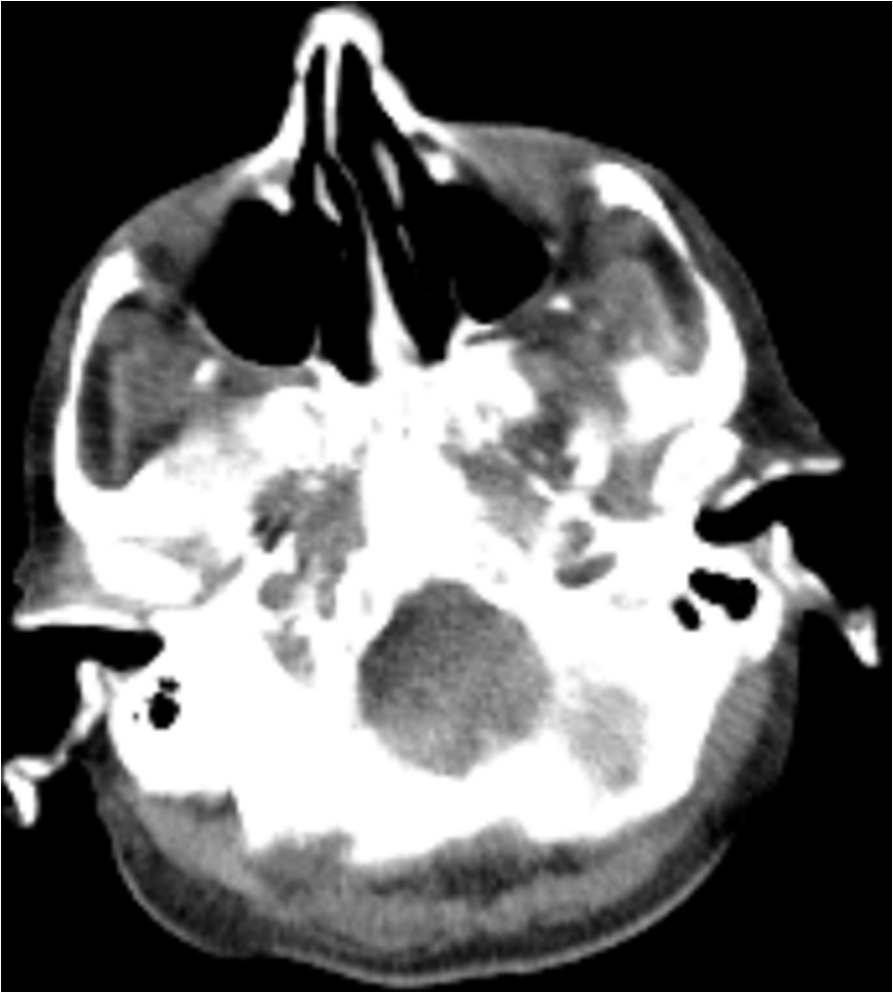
Computed tomography scan of the cerebrum indicates a fully calcified external auditory canal.
